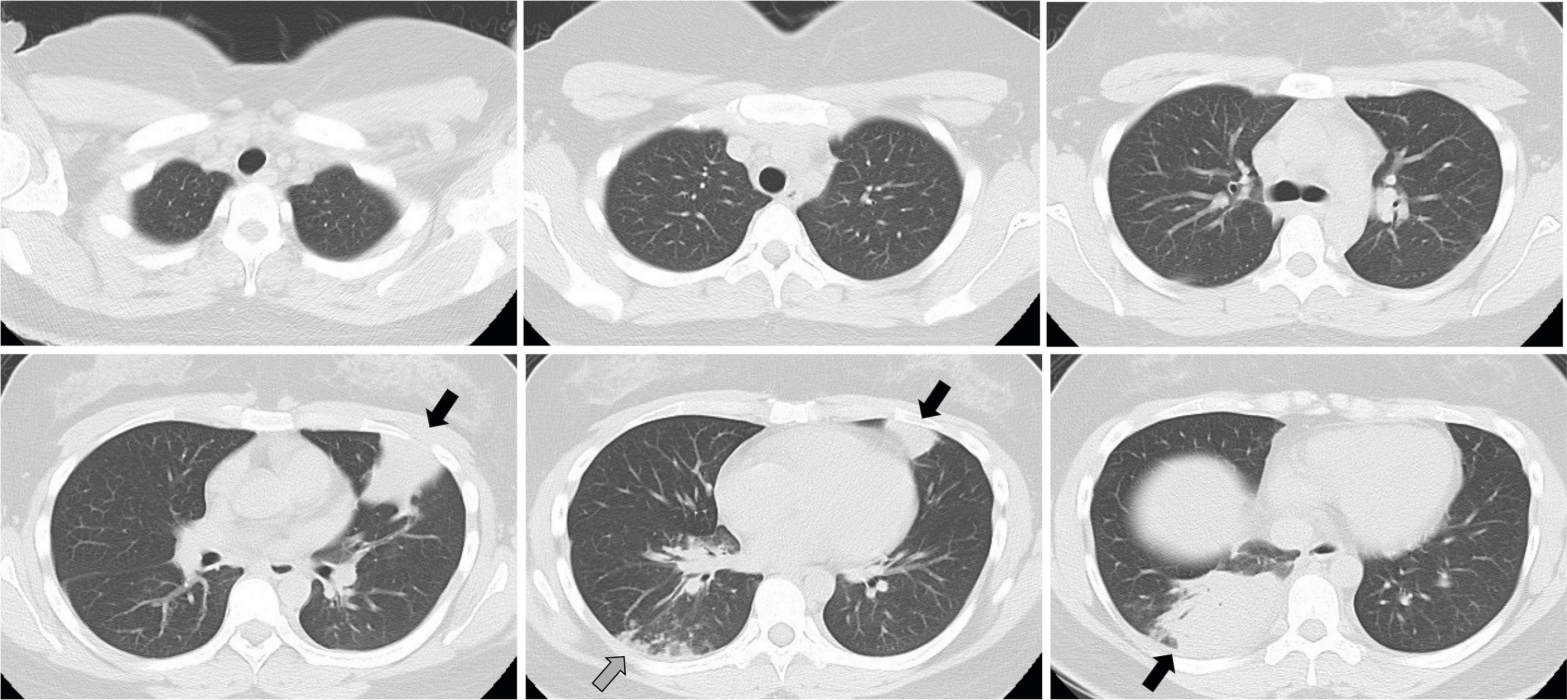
Chest computed tomography five days prior to admission showing centrilobular nodules, bronchial wall thickening (gray arrow), and consolidation in the right lower lobe and left lingular segment (black arrows)
